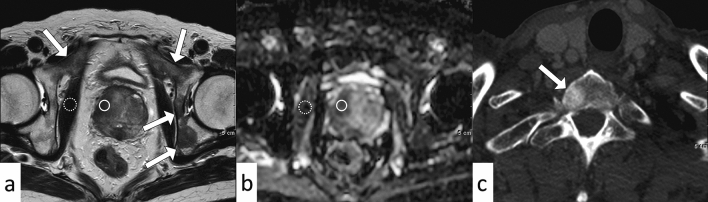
A 79-year-old man with prostate cancer with osteoblastic bone metastases (prostate-specific antigen density = 12.9). Prostate MRI shows the primary tumor (Gleason score 8) with a normalized T2 signal intensity of 2.7 (a, T2 signal intensity of prostate cancer (circle), divided by the T2 signal intensity of internal obturator muscle (dotted circle)), and normalized apparent diffusion coefficient of 0.67 (b, mean ADC of PCa (circle), divided by the ADC of the internal obturator muscle (dotted circle)). Multiple bone metastases are shown on the T2-weighted image (a, arrows). Axial CT reveals an osteoblastic lesion that is indicative of a bone metastasis (c, arrow).
